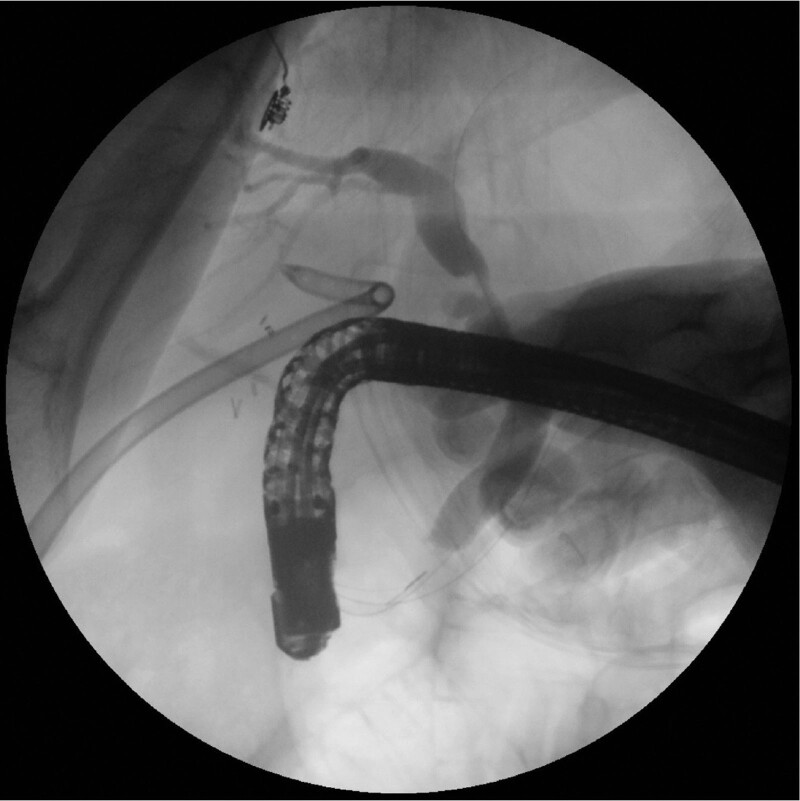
Radiographic view of conventional ERCP performed after antegrade passage of the duodenoscope through the laparoscopic port. ERCP = endoscopic retrograde cholangiopancreatography.
